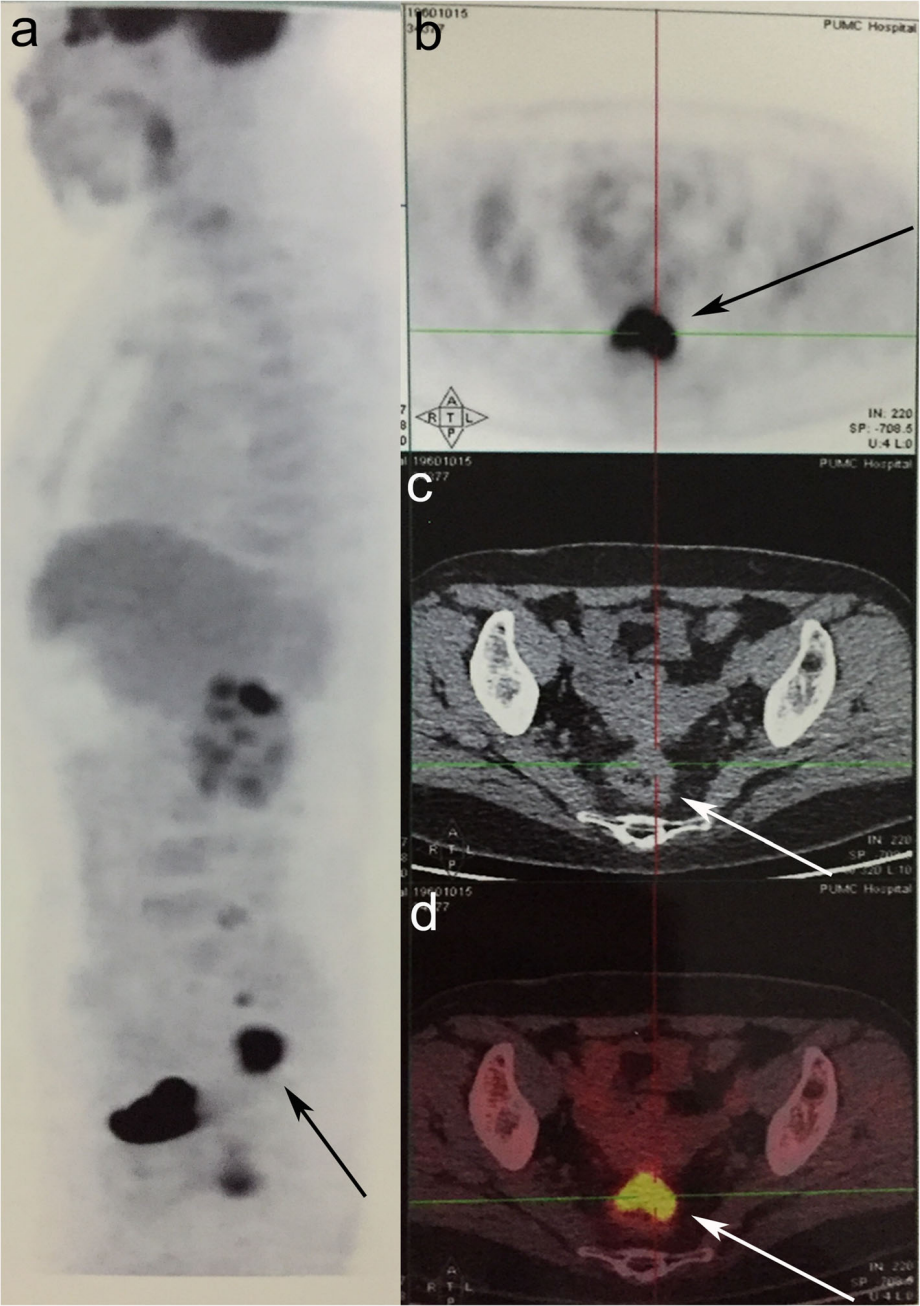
True positive focal-fluorodeoxyglucose (FDG) colonic activity in a positron emission tomography/computed tomography (PET/CT). a FDG-PET/CT maximum intensity projection image showing the intensity of a hypermetabolic wall thickening in the recto-sigmoid colon (maximum standardized uptake value of 15.7). b FDG images (c) CT images and (d) hybrid images of the malignancy
